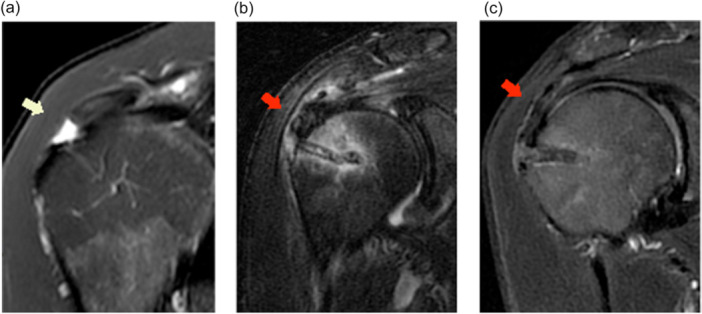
MRI imaging. (a) Preoperative MRI of the patient shoulder with a complete supraspinatus tear with minimal retraction (yellow arrow); (b) 2‐day postoperative MRI of the same patient showing the supraspinatus repair with the decellularized dermal graft lining on the top (red arrow); (c) 6‐month postoperative MRI of the same patient showing the repaired tendon with the decellularized dermal graft still present (red arrow) and partially integrated with native tendon. MRI, magnetic resonance imaging.
